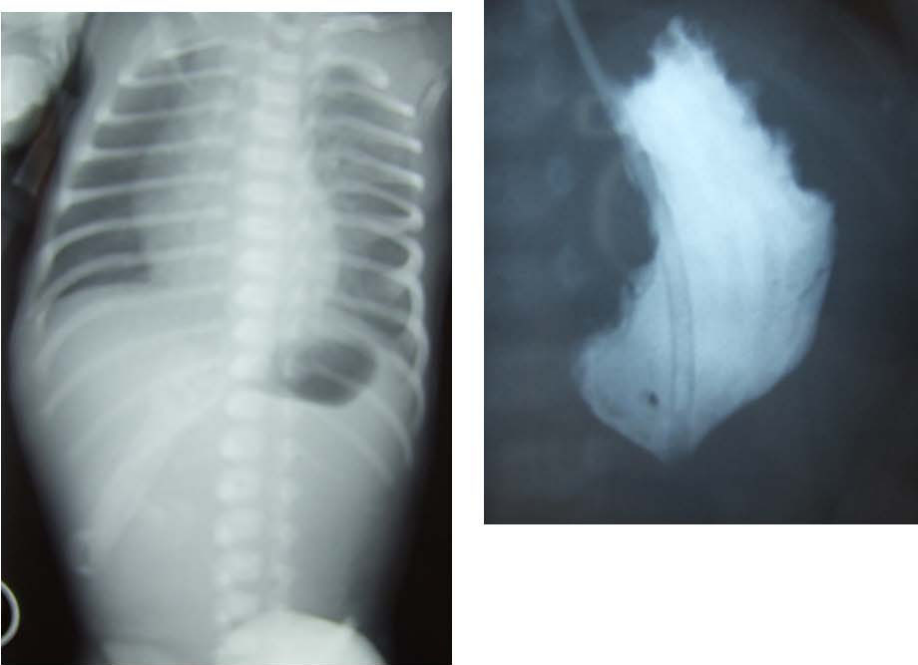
Pre-operative abdominal X-Ray (left) and contrast study with barium meal (right).
